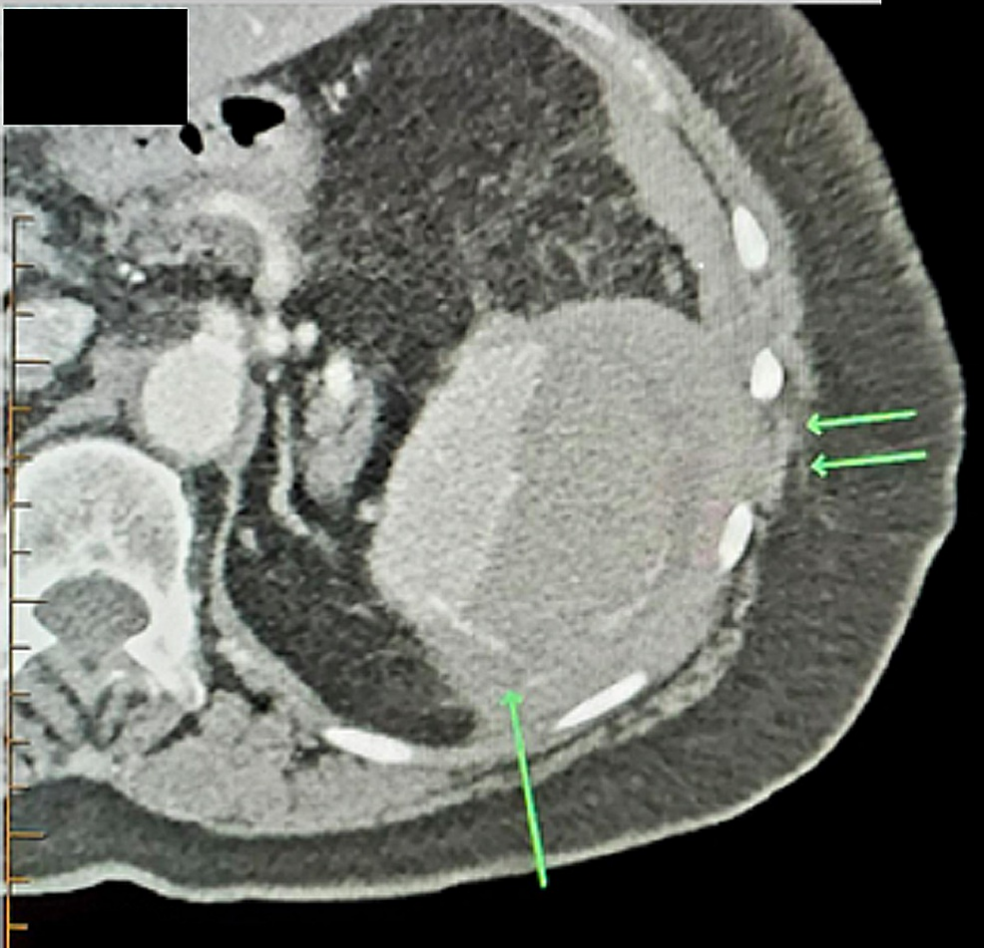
CT abdomen/pelvis of the patient’s spleen The image shows active extravasation (single arrow) and hematoma (double arrow) CT: computed tomography
